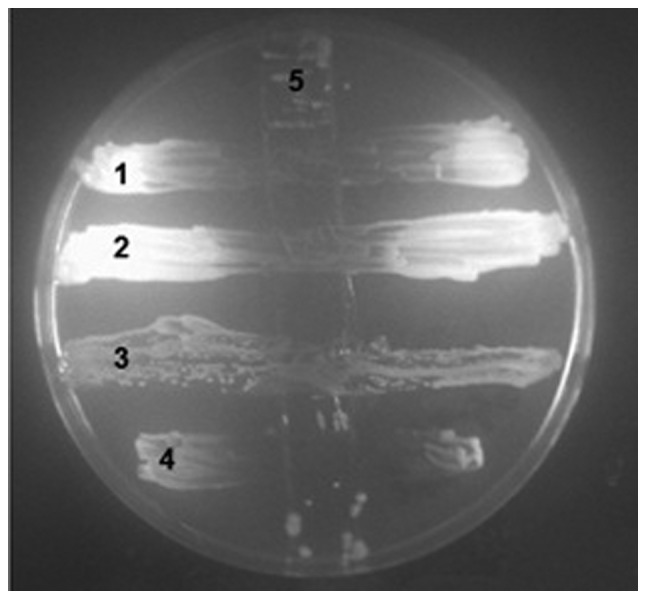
Cross-streak method was performed on 1, CA (ATCC 90028); 2, CT; 3, CG; 4, CP; 5, PA1206 following killing by chloroform. CA, *Candida albicans*; CT, *Candida tropicalis*; CG, *Candida glabrata*; CP, *Candida parapsilosis*; PA, *Pseudomonas aeruginosa*; ATCC, American Type Culture Collection.
